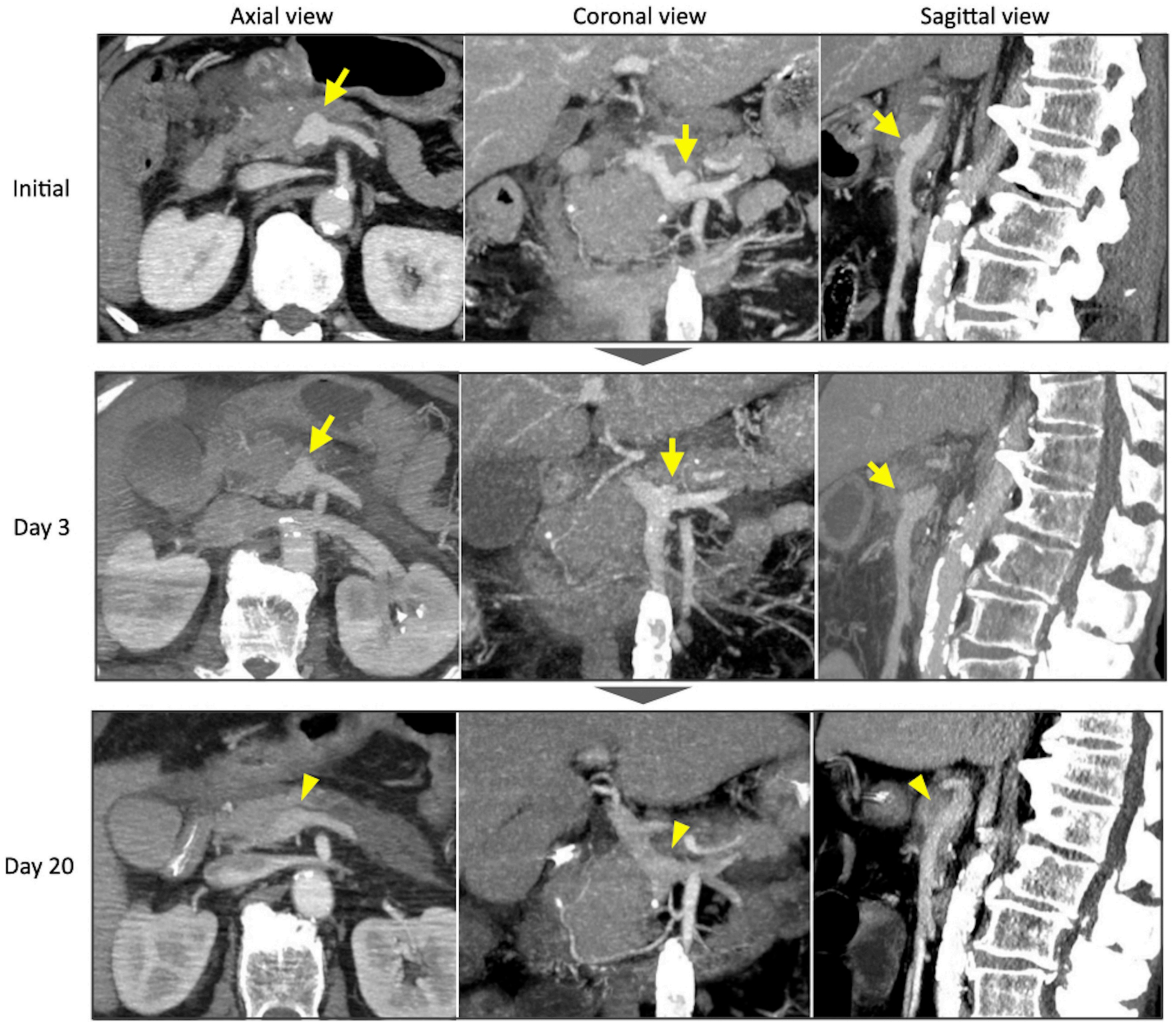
Progress images of portal vein pseudoaneurysm (arrow) detected by maximum intensity protection images from the portal venous phase of contrast-enhanced computed tomography. The pseudoaneurysm diameter normalized 20 days later (arrowhead).
